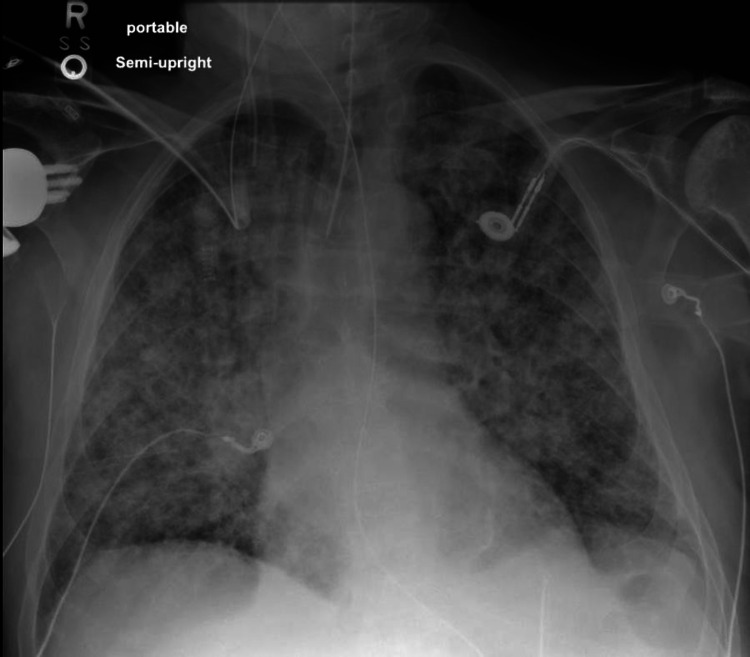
Chest x-ray showing diffuse bilateral pulmonary opacities suggesting the development of ARDS ARDS: acute respiratory distress syndrome
